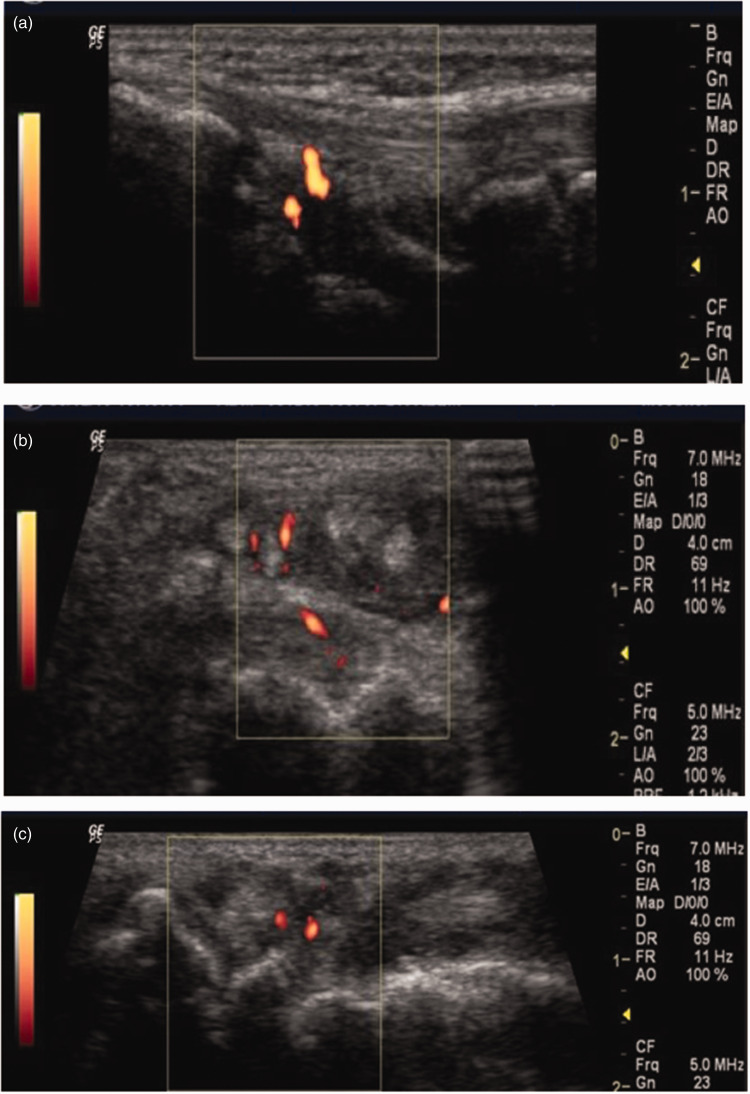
(a) Dorsal longitudinal scan of the right wrist joint using 7–13 MHz linear transducer showing synovitis with positive doppler signal. (b) Transverse dorsal scan showing peri-tendonitis and synovitis grade II doppler signal with irregular cortical surface of the carpal bones (erosive changes) and (c) Dorsal transverse scan showing synovitis, effusion and erosive changes in the cortical surface of the carpal bones.
